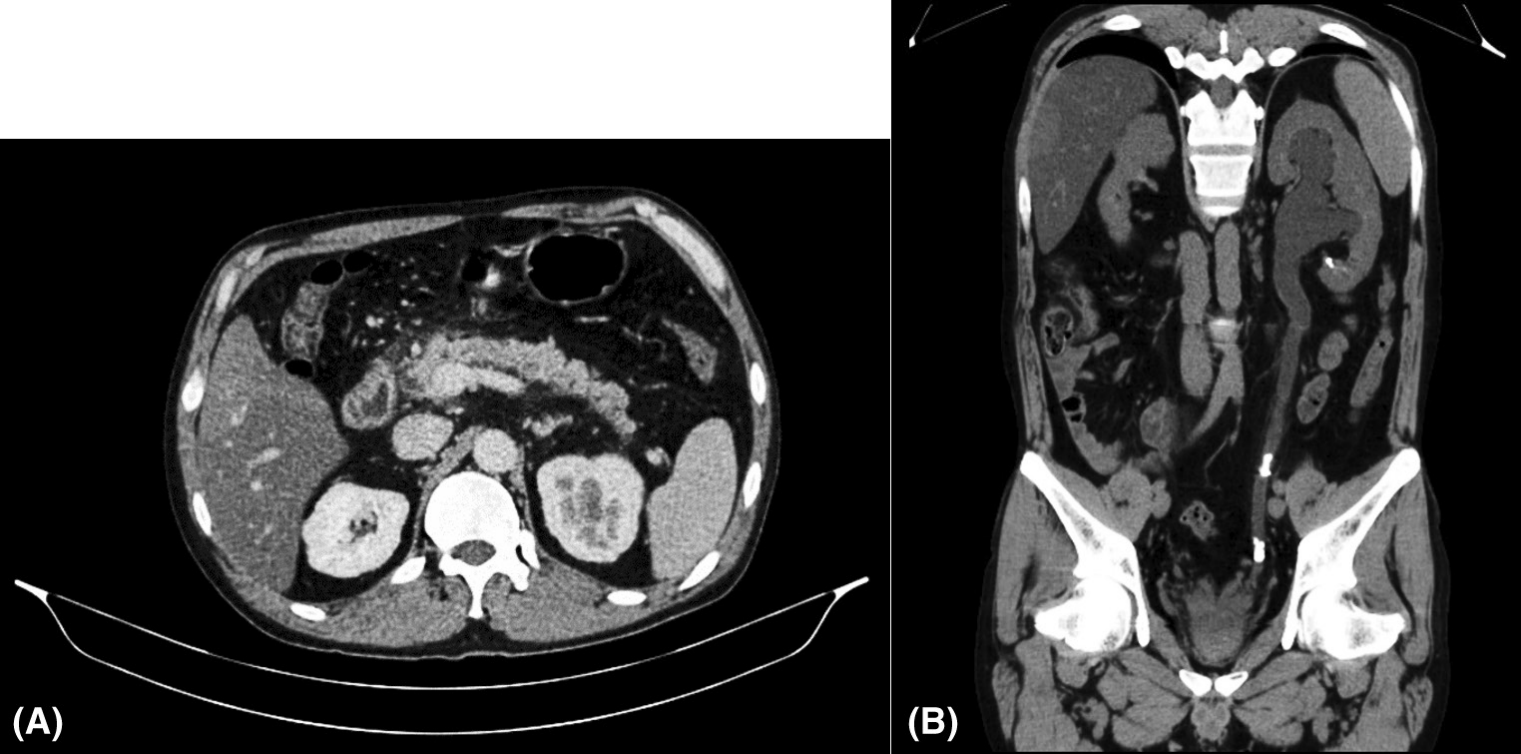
CT scan (Abdomen + Pelvis) suggestive of focal interstitial pancreatitis (A) and left sided ureteric stone (B).
